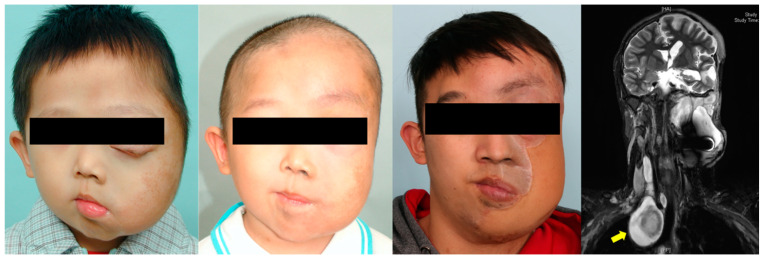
Clinical images of an 11-year-old boy with craniofacial plexiform neurofibromatosis who has been followed up with since the age of 2 and has been receiving orbital and zygomatic repositioning and reconstruction of the orbital roof and temporal bone. Owing to the disease progression, a radical excision was performed after preoperative embolization (branches of the left internal maxillary, lingual, and facial arteries) and total interruption of the left external carotid artery. The soft tissue defect was reconstructed with an anterior lateral thigh flap. Magnetic resonance imaging showed involvement of the left intraorbital, parasellar, parapharyngeal, and posterior pharyngeal regions and adjacent disorganized brain tissues and dural ectasia; craniotomy was performed for skull base and orbital tumor removal. Additional procedures included serial tumor excisions, lip repair, and canthoplasty. Magnetic resonance imaging indicated a mediastinal mass (yellow arrow) with neck swelling, and shortness of breath had developed in recent years. An additional neurofibroma compressing the cervical spine (C2 and C3 level) was also identified.
